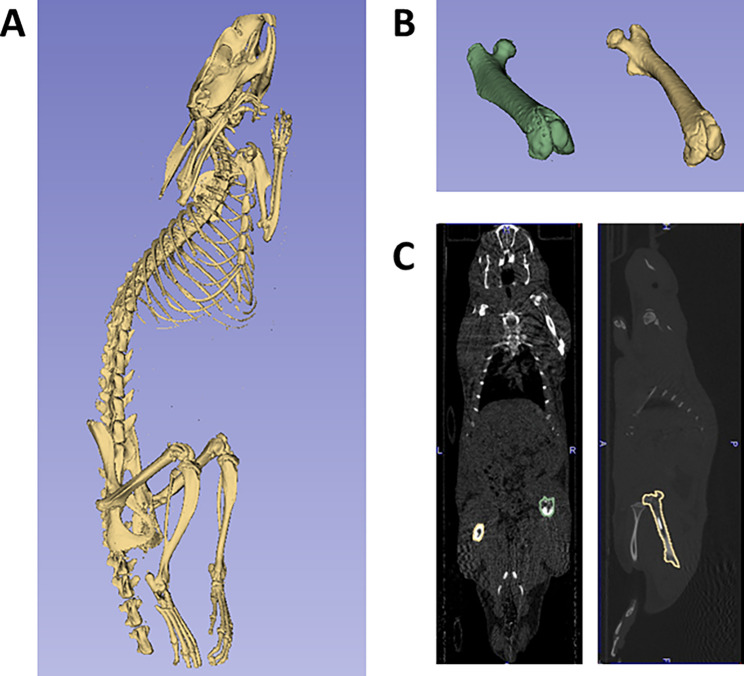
Clear-cut ROIs are used for Standardized Uptake Value analyses. The SUV was normalized to body weight and to the decay of the [^89^Zr]-radioisotope (SUVbw). Calculation of SUVbw was performed by generating specific ROIs. (a) A 3D image of the bone was processed from the CT image after manual thresholding. (b) The femur with a biofilm-infected implant (green) and the femur with a sterile implant (yellow) were manually isolated. The mask-volume option was used to fill in the gaps in both femurs. (c) The posteroanterior and sagittal planes are shown. With clear-cut ROIs, the SUVbw was measured using PMOD software (version 3.910, PMOD Technologies)
